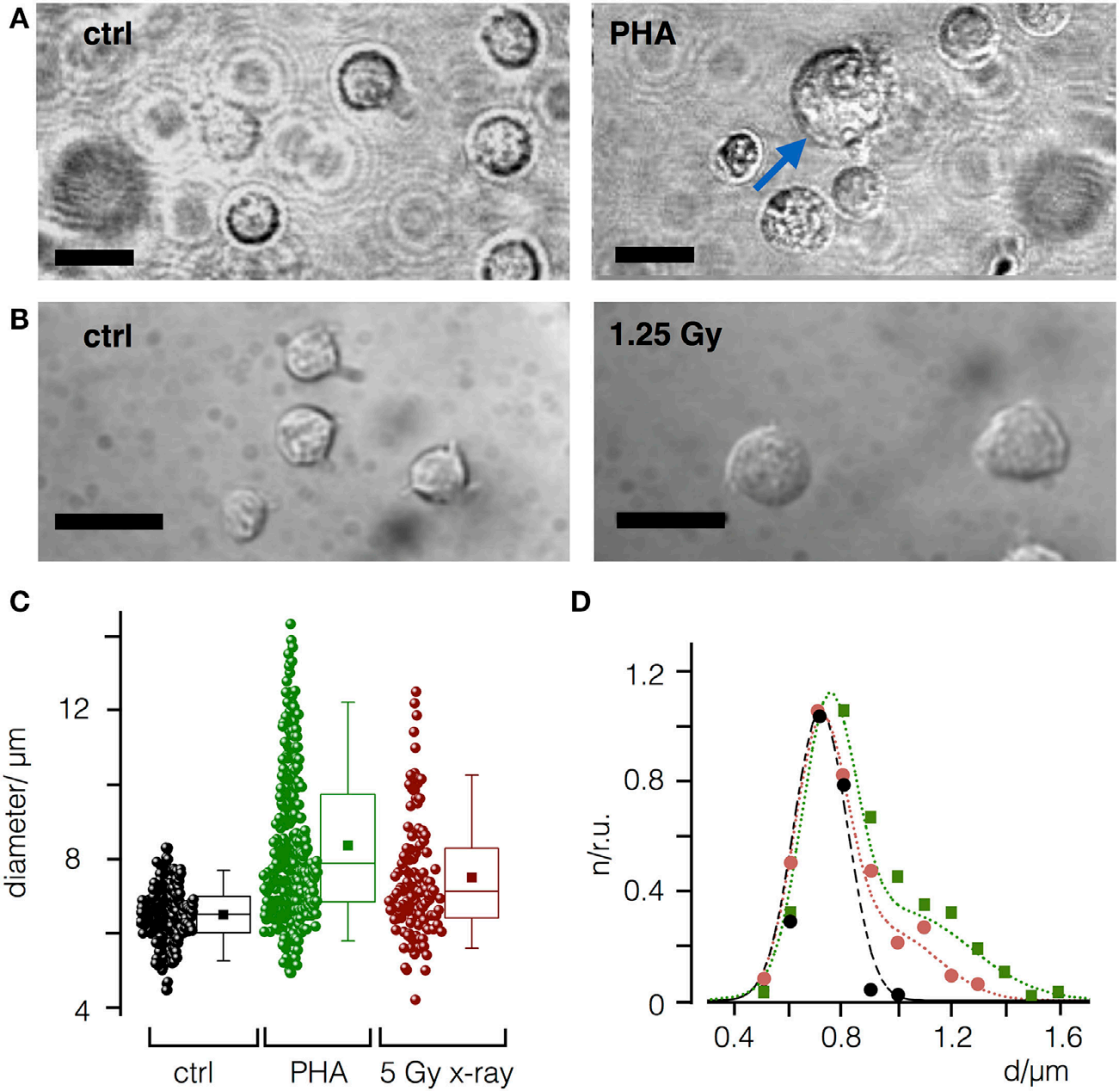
Mitogen phytohemagglutinin (PHA-L) and ionizing irradiation cause an increase in diameter of naïve peripheral blood leukcocytes (PBL). (A) Representative images of PBL before (control) and 48 h after treatment with PHA-L (7.2 µg/m). (B) Before and 48 h after irradiation with a dose of 5 Gy. Scale bars 10 µm. (C) Cell diameters of PBL control cells (ctrl) or PBL treated with PHA-L or 5 Gy. Each data point represents a single PBL with mean (filled square) and median value (line) as well as 25 and 75 percentile of data; whiskers indicate 5 and 95 limits of data. (D) Size distribution histogram of ctrl (black) and of cells treated with PHA-L (green) or 5 Gy (red) from (B). Distribution was normalized to maximal value for each condition and fitted with single Gauss distribution (control, black line) or the sum of two Gaussians for T-cell activator (green line) or X-ray (red line) treated cells.
